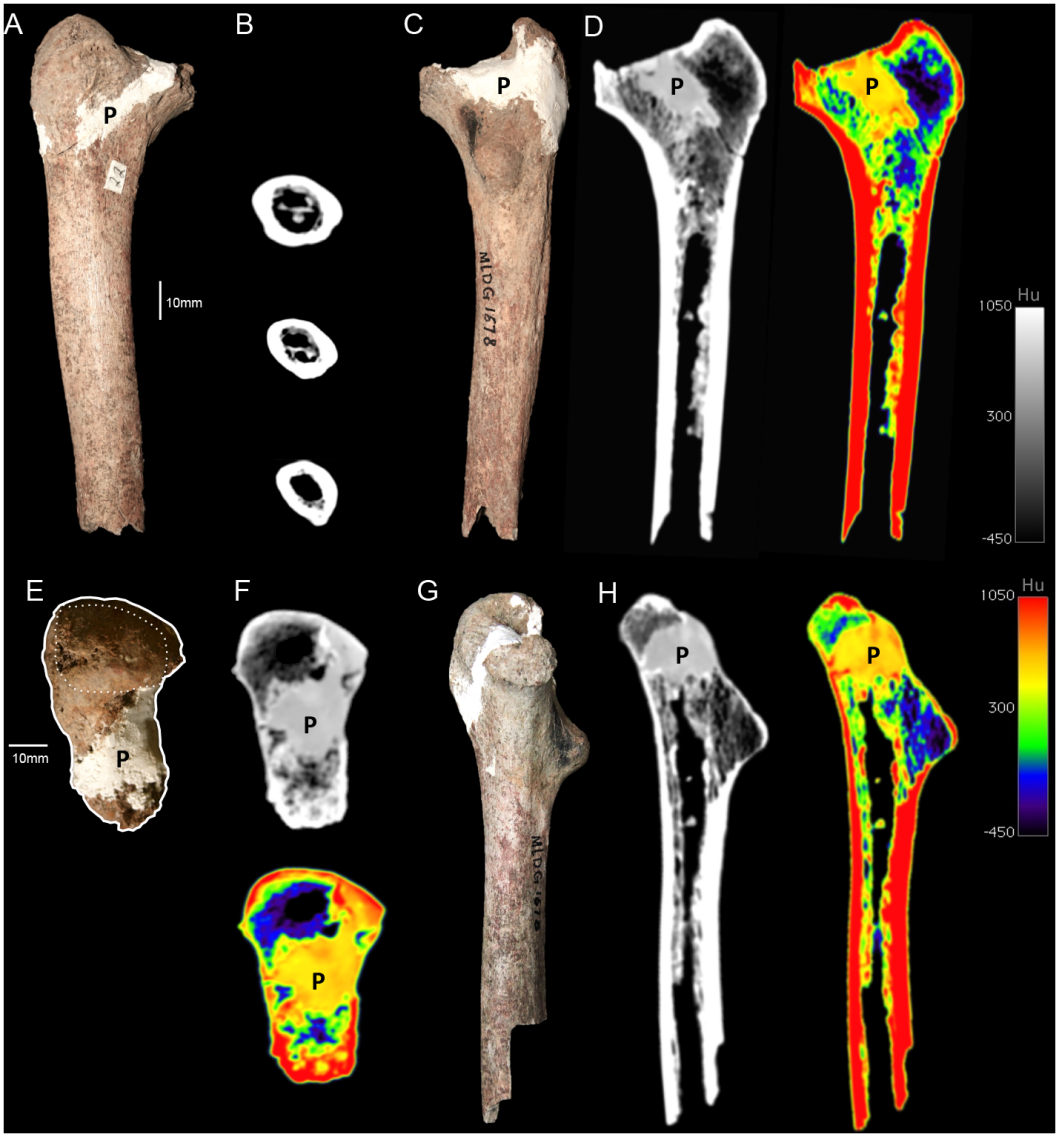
Femur MLDG 1678: (A) Anterior view. (B) CT-scan slices at subtrochanteric, approximate half-way and mid-shaft levels. (C) Posterior view. (D) CT-scan slice at approximately mid-coronal plane, grayscale (left) and colour density map (right). (E) Superior view highlighting the overall outline and superior surface of the greater trochanter (anterior at left, lateral at top). (F) CT-scan transverse slices at approximate mid-neck level, grayscale (left) and colour density map (right). (G) Medial view. (H) CT-scan slices in approximate mid-plane, grayscale (left) and colour density map (right). (P = plaster added in 1989 during restoration).
